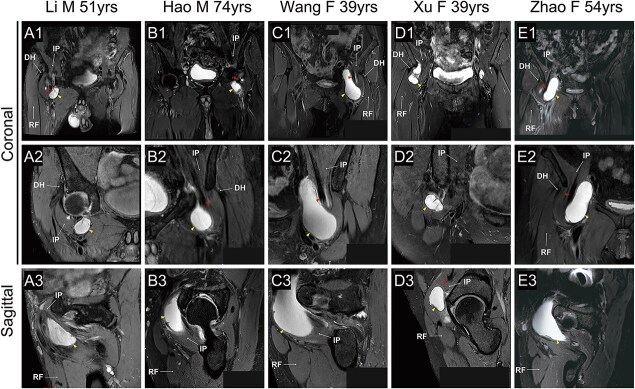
MRI for iliopsoas cysts. Iliopsoas tendon (red arrowhead); iliopsoas cysts (yellow arrowhead); IP, iliopsoas; DH, the direct head of rectus femoris; RF, rectus femoris; M, male; F, female.
